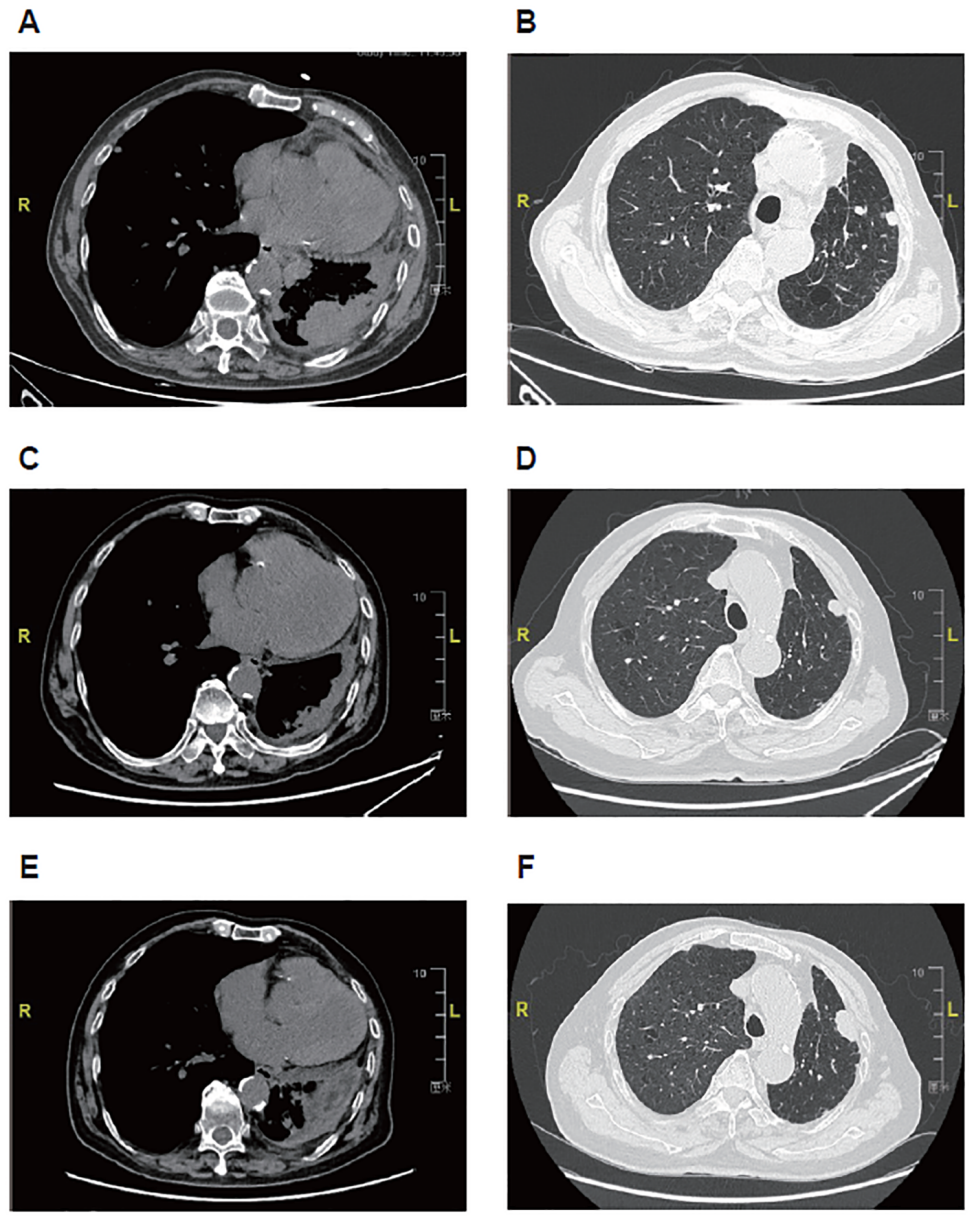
Representative images of computed tomography in an 81-year-old male patient with metastatic non-small cell lung cancer harboring *HER2* exon 3 T126A mutation and amplification treated with first-line trastuzumab deruxtecan. (A, B) Before treatment of trastuzumab deruxtecan. (C, D) After 12-month treatment of trastuzumab deruxtecan. (E, F) After 16-month treatment of trastuzumab deruxtecan.
